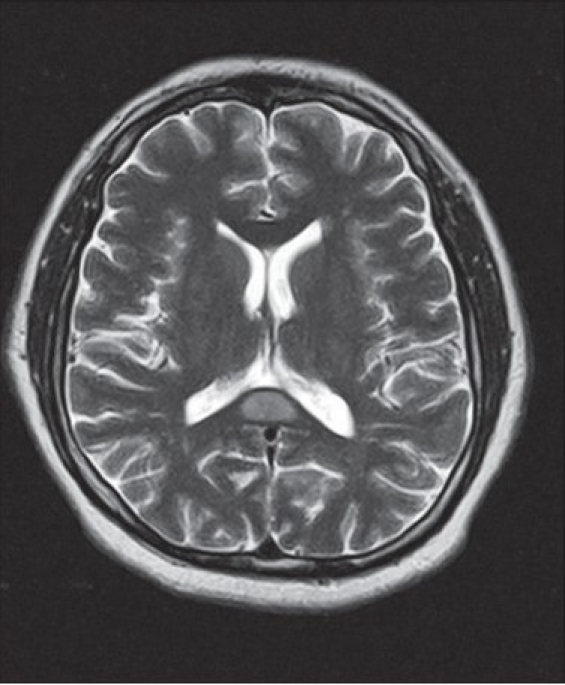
Axial T2-weighted sequence shows that the lesion is homogeneously hyperintense compared to the body of the corpus callosum
